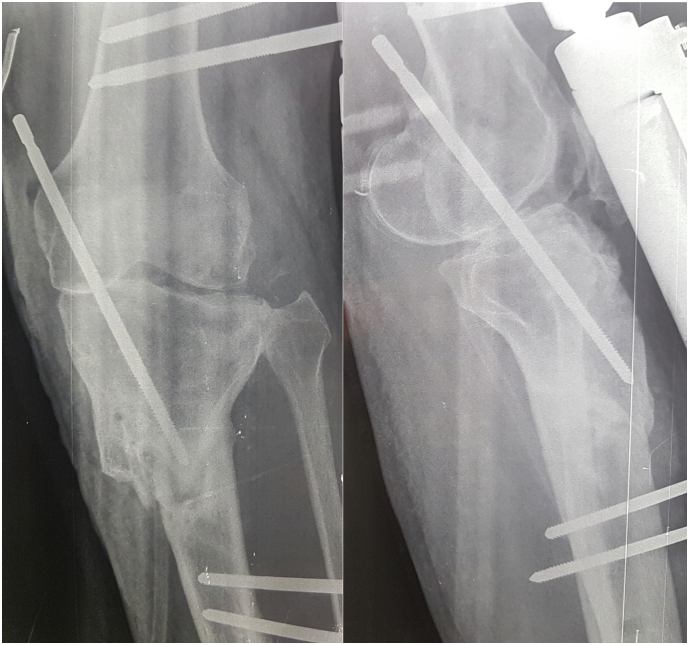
Knee radiographs showing reduction of joint and fixation with orthofix external fixator and an intra-articular Steinman pin.
